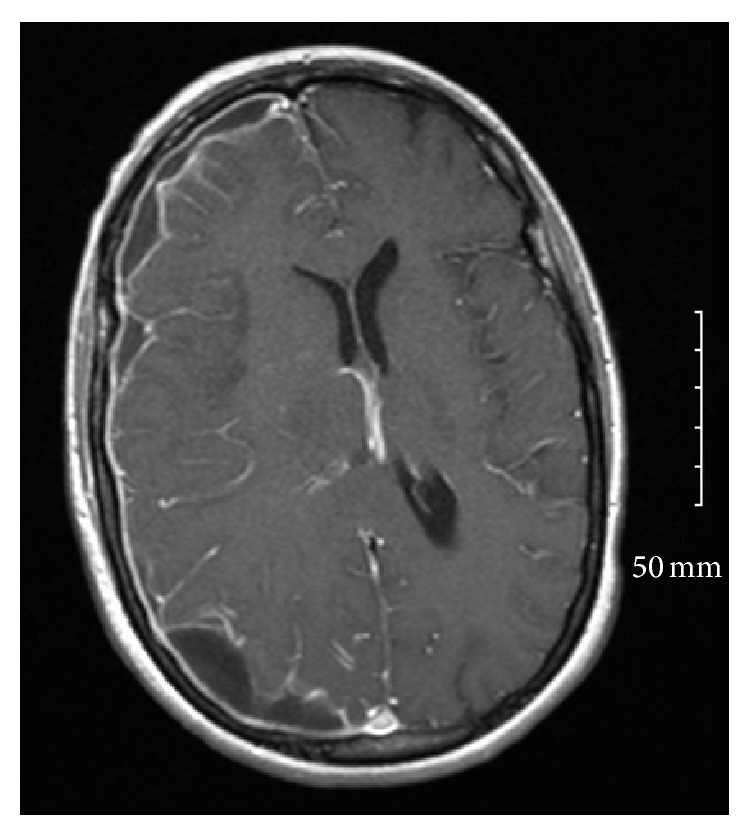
T1 MRI image demonstrating complicated right hemispheric subdural empyemas and right to left midline shift.
